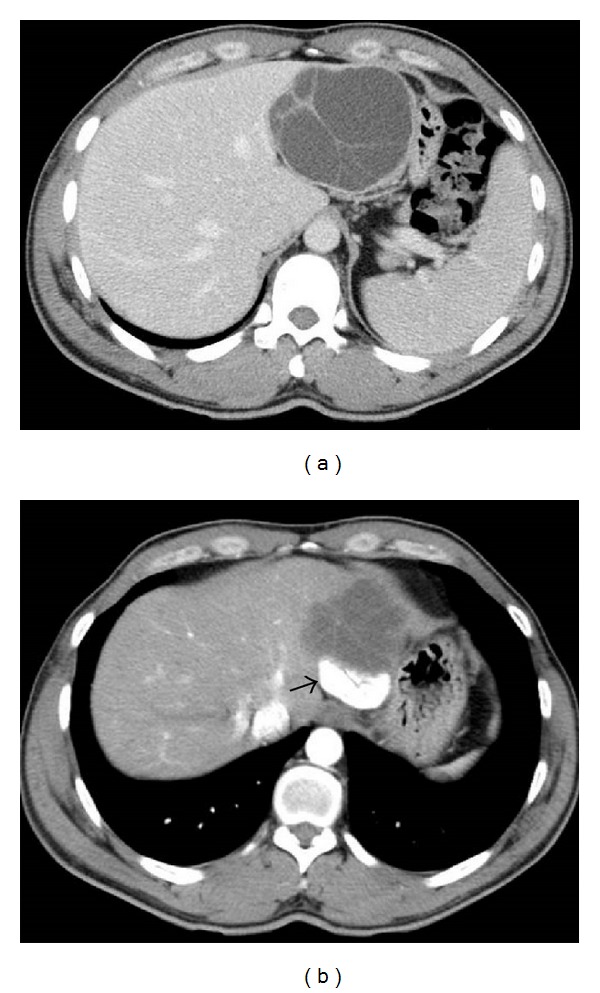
Enhanced computed tomography of the abdomen revealed a large cystic lesion, 9 cm in diameter, in the lateral segment of the liver, which has many honeycomb-like septa (a) with calcified lesions (b arrow).
